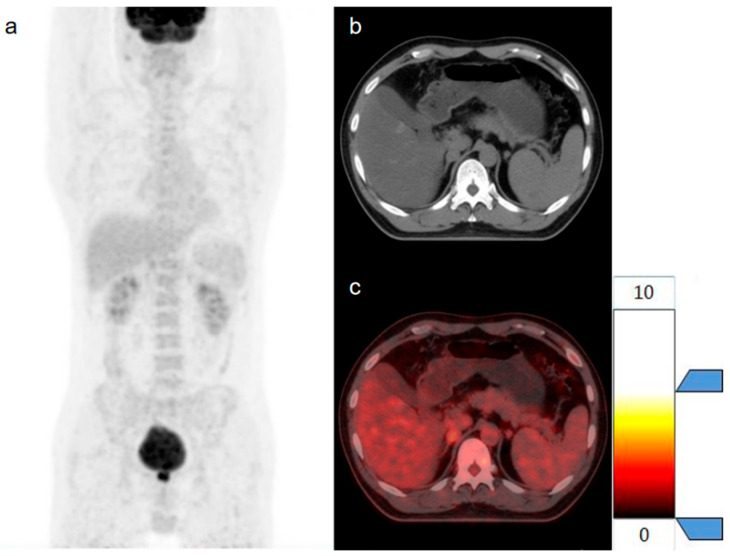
Patient No. 15 (a) The maximum density projection (MIP), (b) the high-resolution CT, and (c) the PET/CT.
